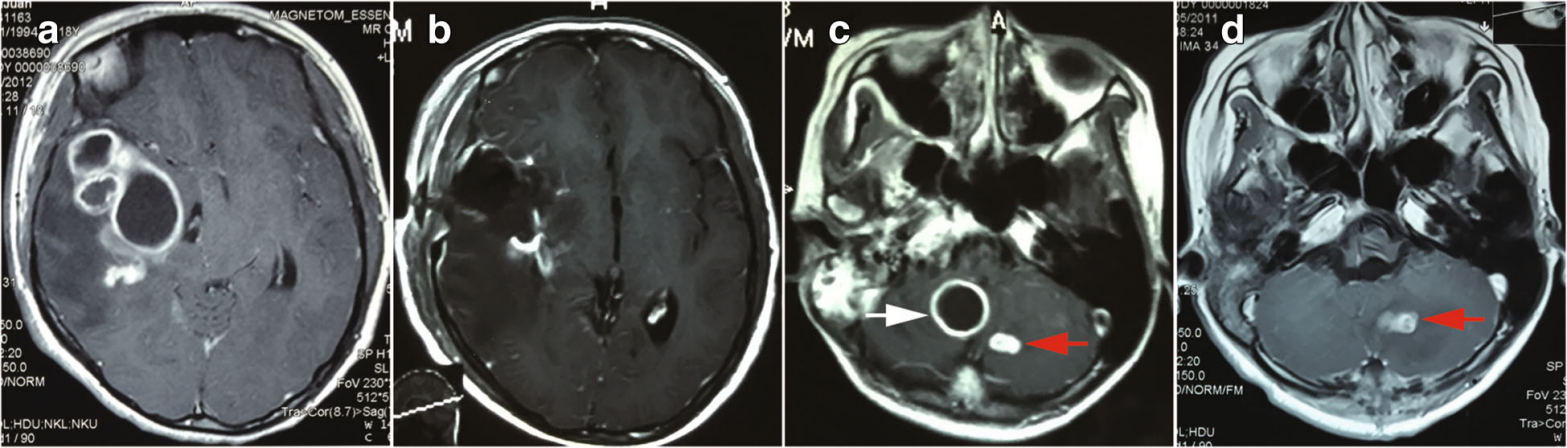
Pre and post-surgical image of intracisternal tuberculoma. a Preoperative enhancing MRI of a tuberculoma locating at right sylvian fissure. b Postoperative enhancing MRI of a tuberculoma locating at right sylvian fissure. c Preoperative enhancing MRI showed a tuberculoma locating at right cisterna magna (white arrow). The tuberculoma of left cerebellum (red arrow) was resolved by ATT. d Postoperative enhancing MRI showed the tuberculoma locating at right cisterna magna was excised. The tuberculoma of left cerebellum (red arrow) was resolved by ATT in later period
